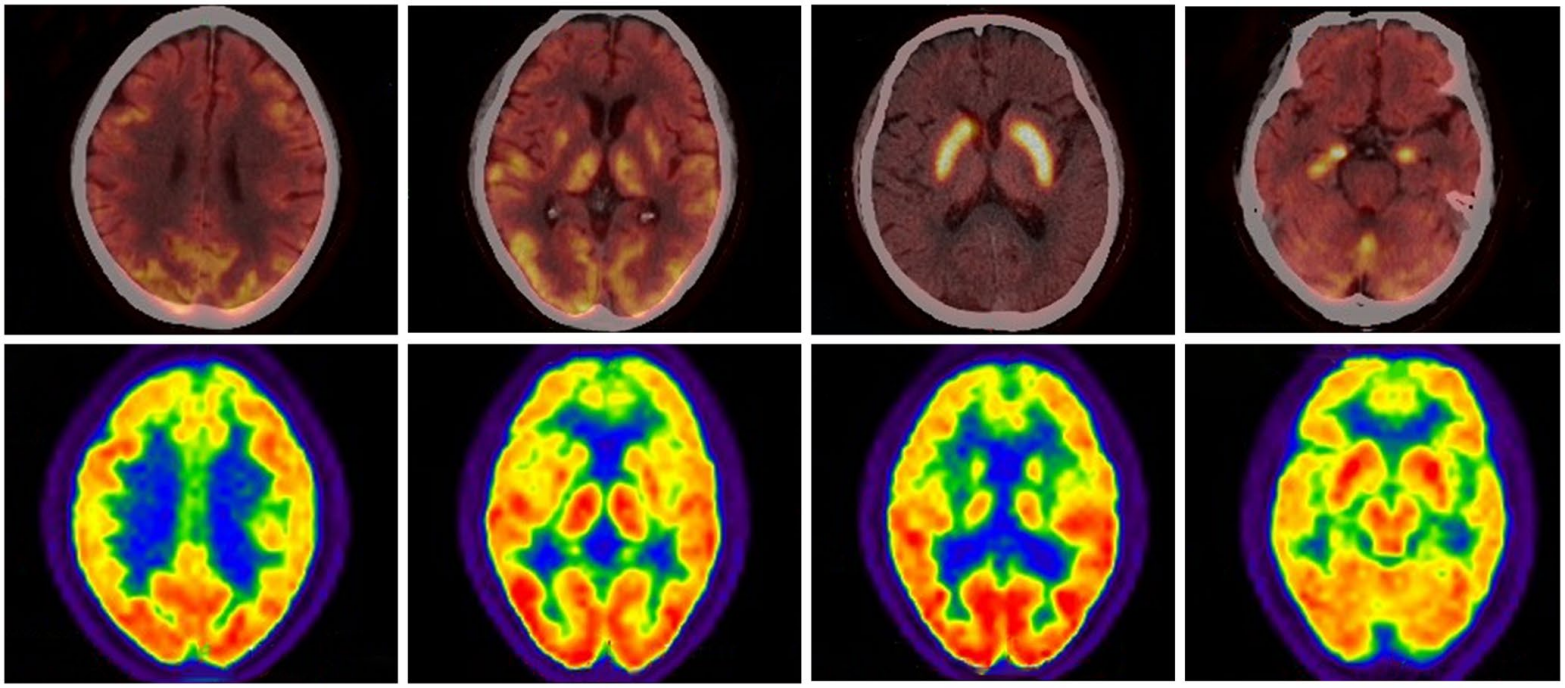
Bilateral temporal hypermetabolism on 18F‐FDG PET in LGI1‐AE. 18F‐FDG PET demonstrates bilateral hypermetabolism in the deep temporal lobes adjacent to the hippocampus.
